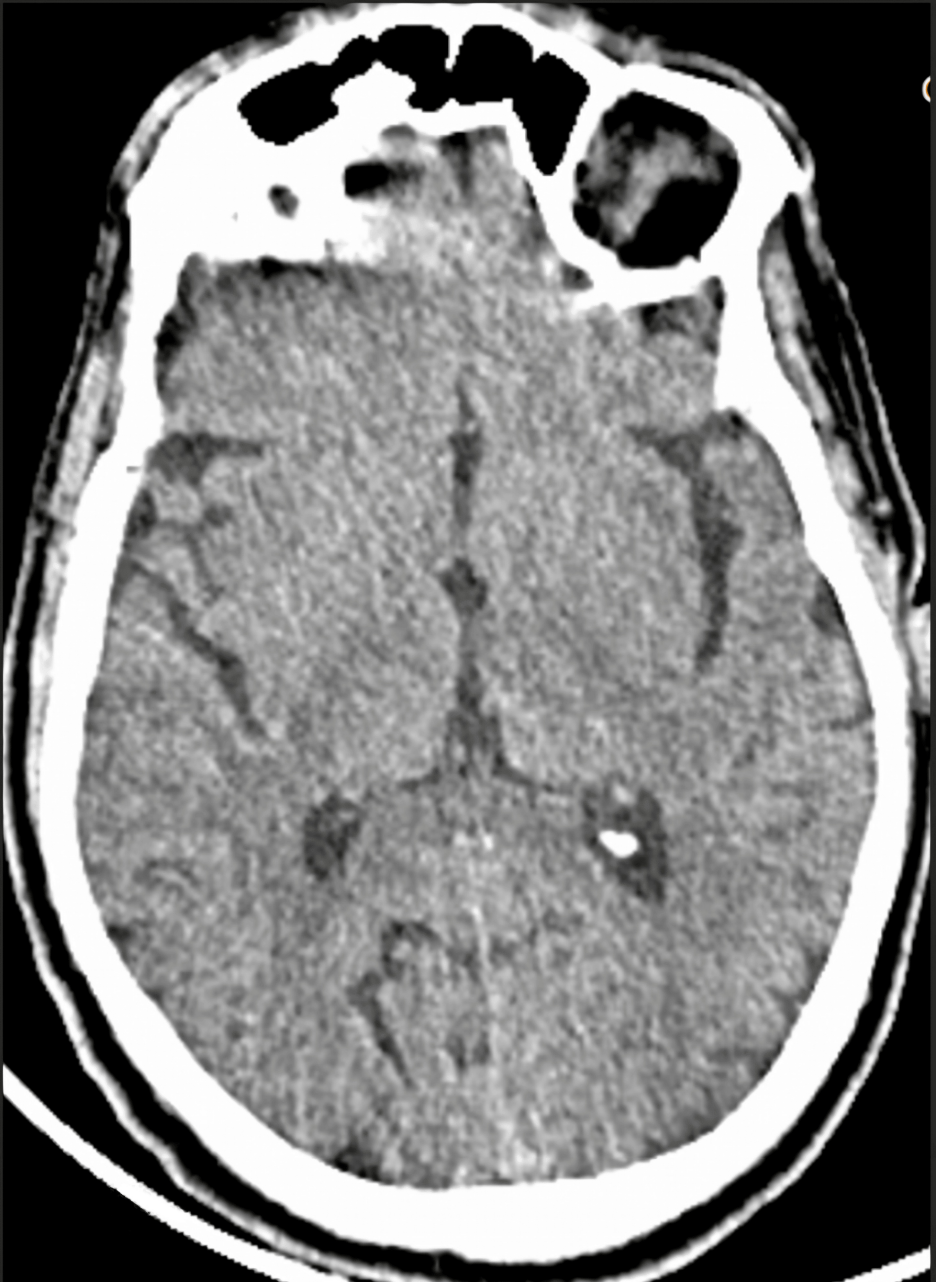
Normal baseline non-enhanced CT scan, without signs of hemorrhage or acute infarction.
